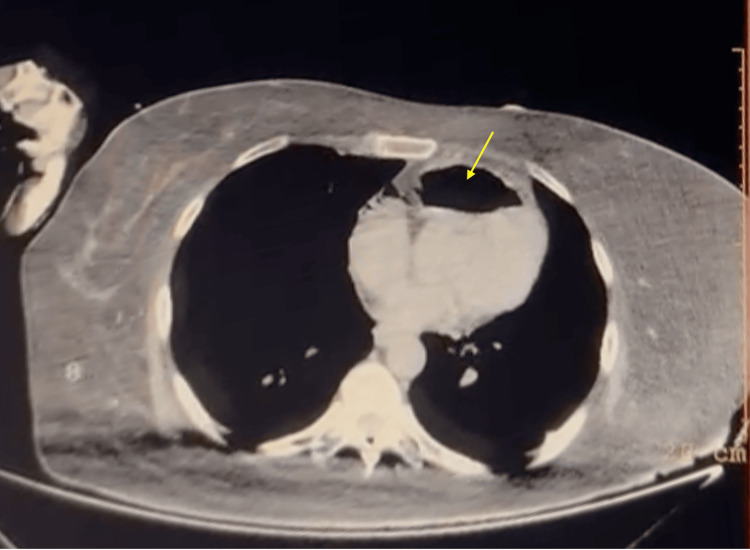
Non-contrast CT of the chest (axial view) showing a distinct radiolucent area (yellow arrow) within the right ventricle, consistent with the presence of air embolism.
